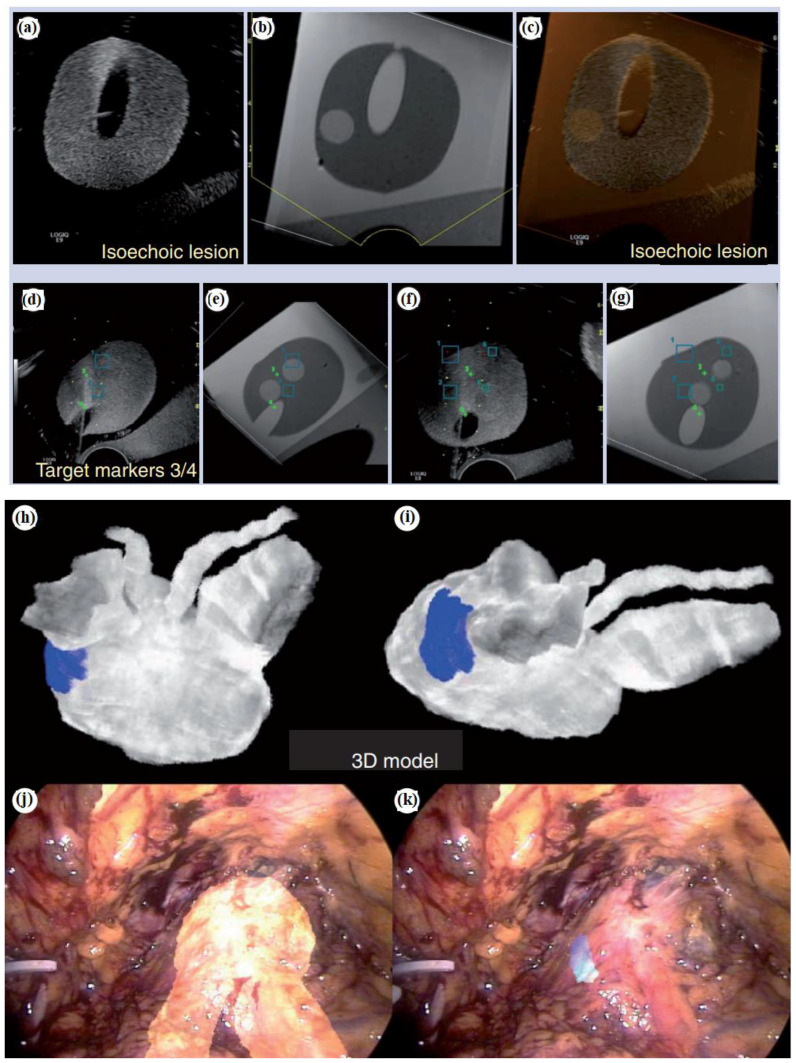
Fusion platform for hybridizing transrectal ultrasonography (TRUS) with magnetic resonance imaging (MRI). (a) For axial TRUS image in real-time mode, there is no detectable lesion. (b) The lesion on the right side is visualized with the synchronized, previously acquired MR image. (c) The MR overlays onto the TRUS as image fusion to facilitate the determination of the lesion which is visible only on the MR. (d) Real-time TRUS visualization of the hyperechoic needle (with a GPS-like needle tracking system) documents with spatial digital data the precise location of each biopsy sample. (e) Digital marks are used to register the spatial coordinates of both the distal and proximal endpoints of the biopsy trajectory. (f) Subsequently, for spatial re-targeting, real-time TRUS guidance is employed. (g) With GPS-like computer-based marking of the previous biopsy position, biopsy location is determined using newly acquired 3D volume data (1). Augmented reality navigation in laparoscopic radical prostatectomy: (h) and (i) show views from different angles. In order to determine the blue-colored cancer area (which is proved with biopsy) on the left-posterior-lateral surface of the prostate, intraoperatively, the workstation reconstructed a 3D prostate surgical model. (j) The 3D model of the prostate and seminal vesicles are overlaid onto the real endoscopic view with augmented reality techniques. (k) The cancer lesion is overlaid on the left-posterior-lateral prostate (the 3D model) (1). Reproduced from (1), with permission from Taylor & Francis.
